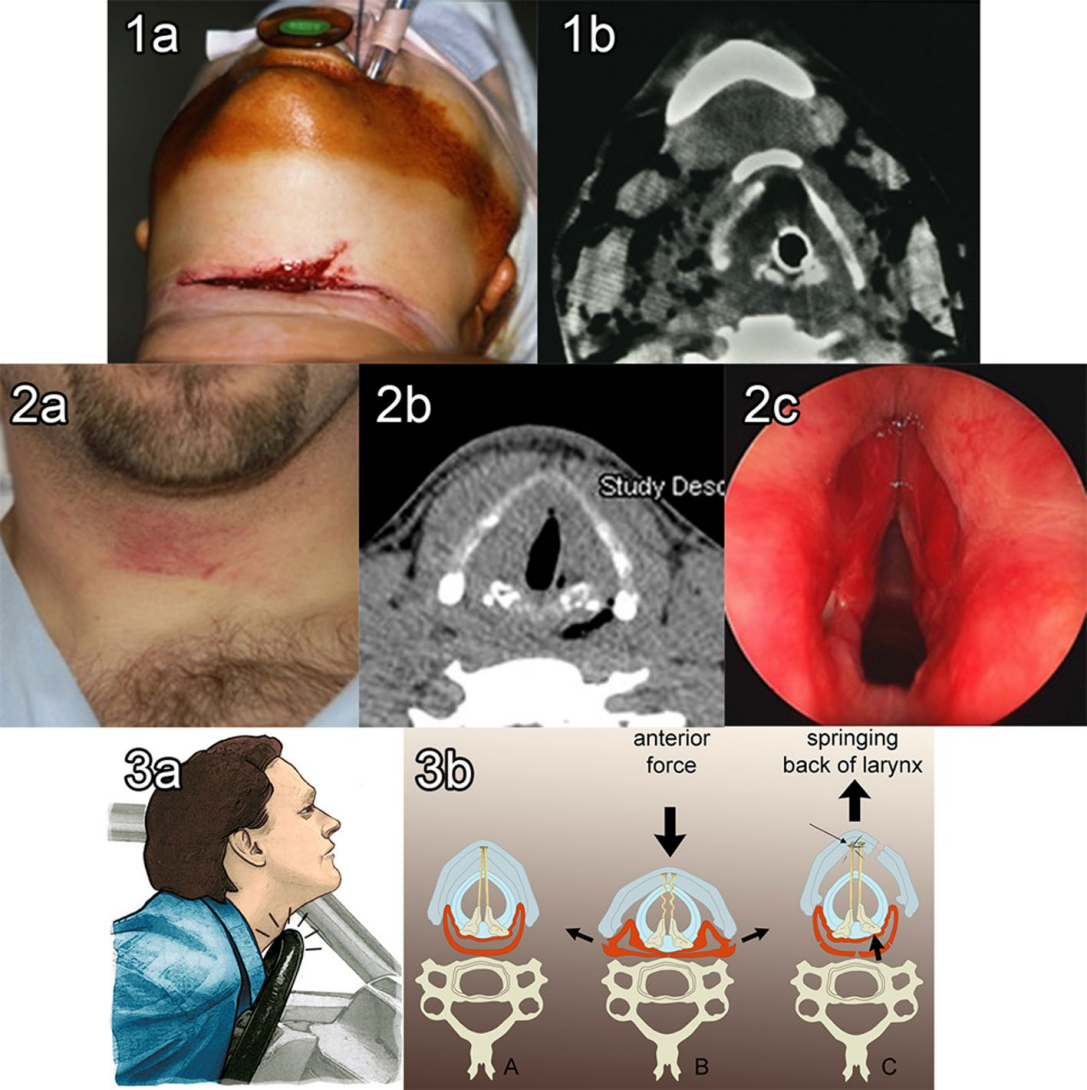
Types of laryngeal injuries. 1a Penetrating injury. 1b CT scan of the intubated patient showed a thyroid cartilage fracture. 2a Blunt trauma injury following a hockey puck injury. 2b CT scan showed an air pocket in the right retro-arytenoid region. 2c Bilateral vocal cords hematoma. 3. Mechanism of blunt laryngeal injury. 3a Crushing forces on the anterior neck due to the steering wheel during deceleration with hyperextension of the neck. 3b [A] Vocal cords and the pharynx in resting state. [B] The anterior force causes median and paramedian thyroid cartilage fractures and pharyngeal lacerations (black arrows). [C] Springing back of the larynx causes arytenoid dislocation and vocal cord(s) disinsertion at the anterior commissure
